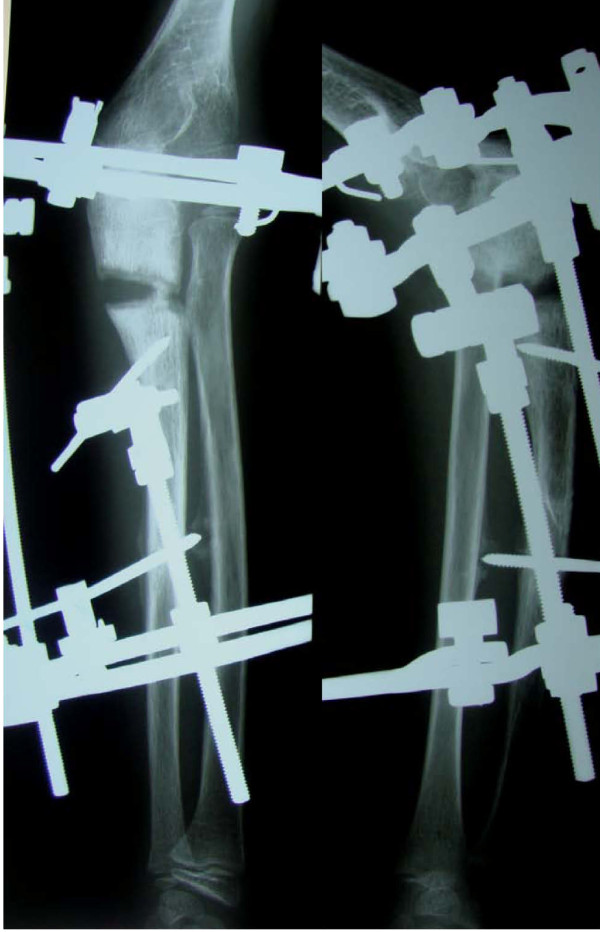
Postoperative radiograph during disraction lengthening (Patient 2).
